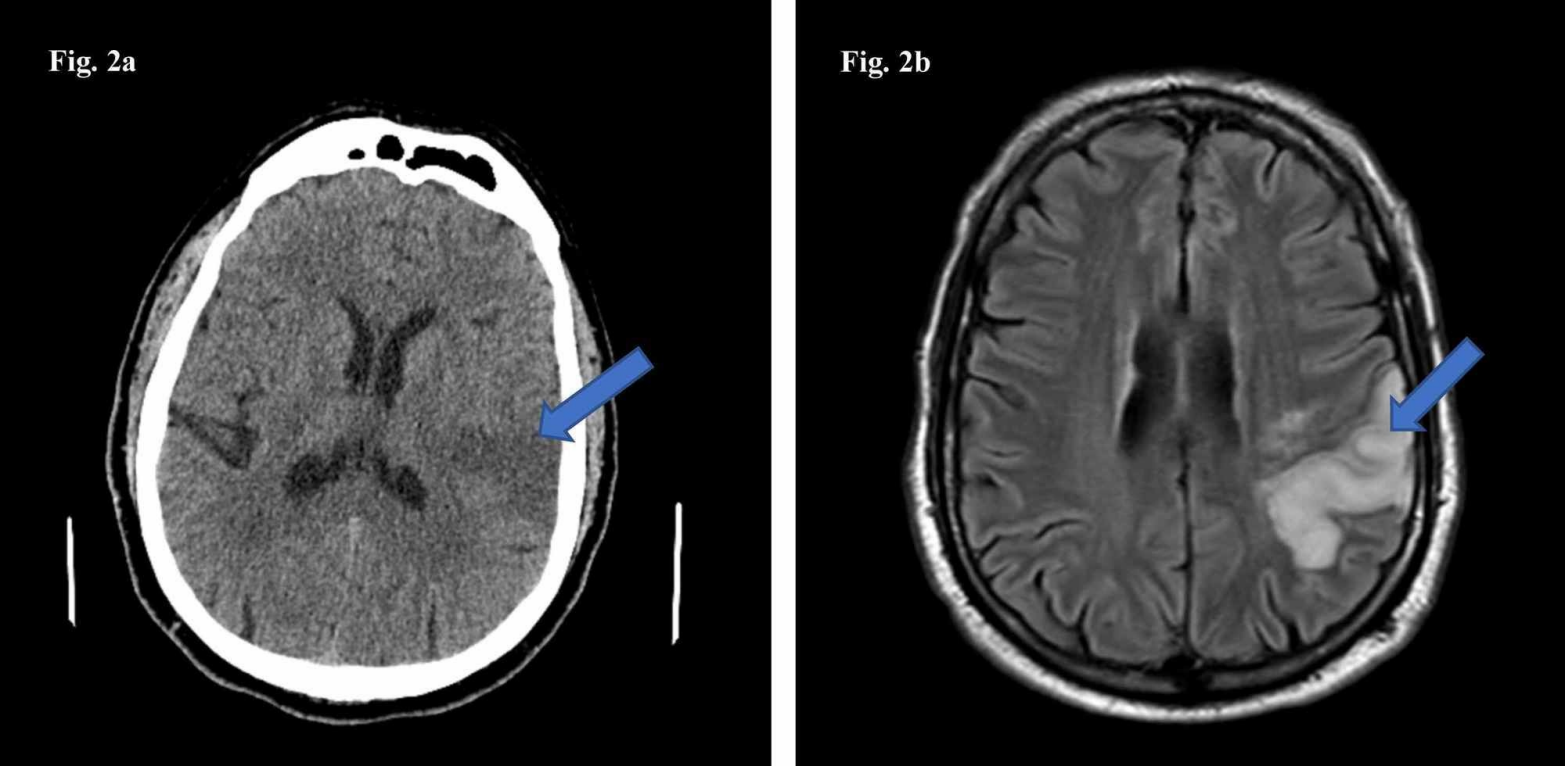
CT scan of the head (2a) and MRI of the brain (2b) showing an evolving moderate-sized subacute left MCA branch infarct (arrows).
